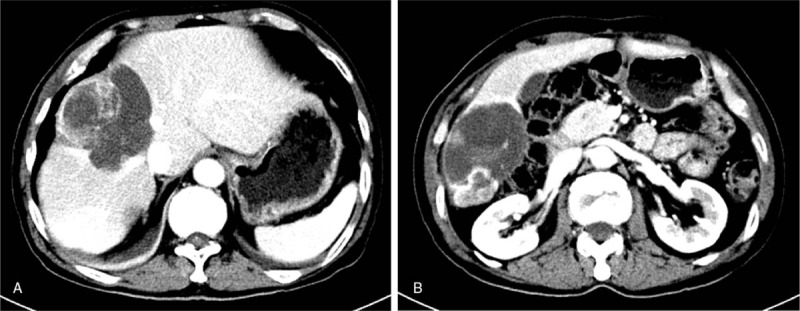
Tumor size on computed tomography (32 months after operation): multiple solid portions of the liver mass were increased. (A) 8.4 × 8.5 cm. (B) 9.1 × 6.3 cm.
